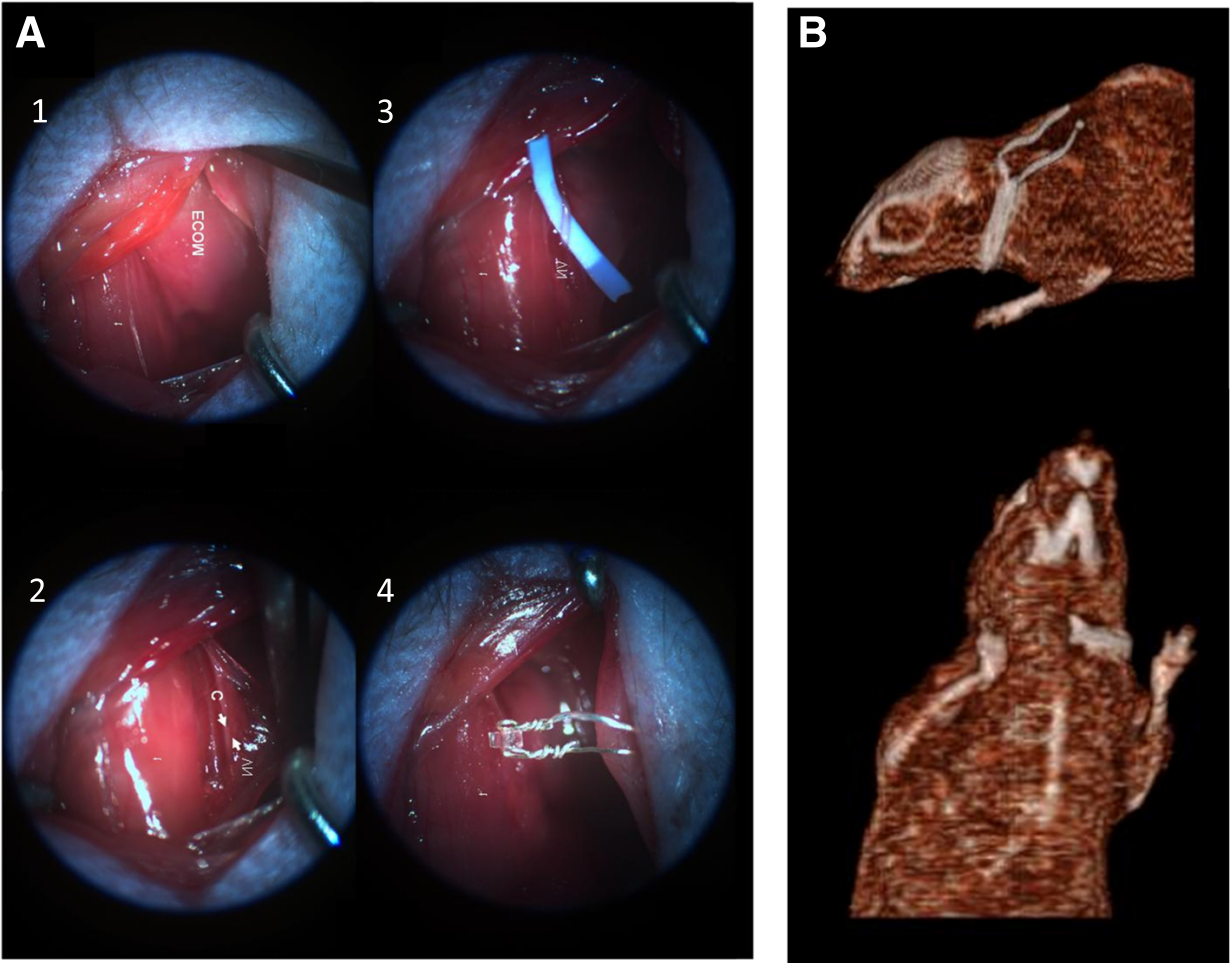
Procedure of implanted electrodes and location by CTscan. a Procedure of electrode insertion into the vagus nerve. 1. Photograph showing the trachea and the sternocleidomastoid muscle, carotid. 2. Sheath with carotid and vagus nerve. 3. Vagus nerve is shown isolated. 4. Electrodes applied to the vagus nerve. t = trachea, ECOM = sternocleidomastoid, c = carotid, VN = vagus nerve. b Representative view of the electrodes inserted in the vagus nerve by Computed Tomography Scan
